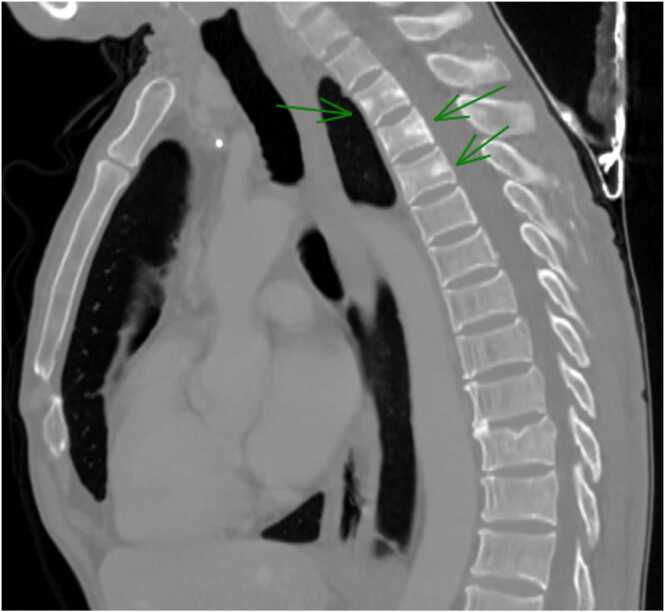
Sagittal section of a contrast-enhanced CT scan showing pseudo-sclerotic bone lesions, hyperdense in the portal phase, involving the vertebral bodies and pedicles from T1 to T3.
